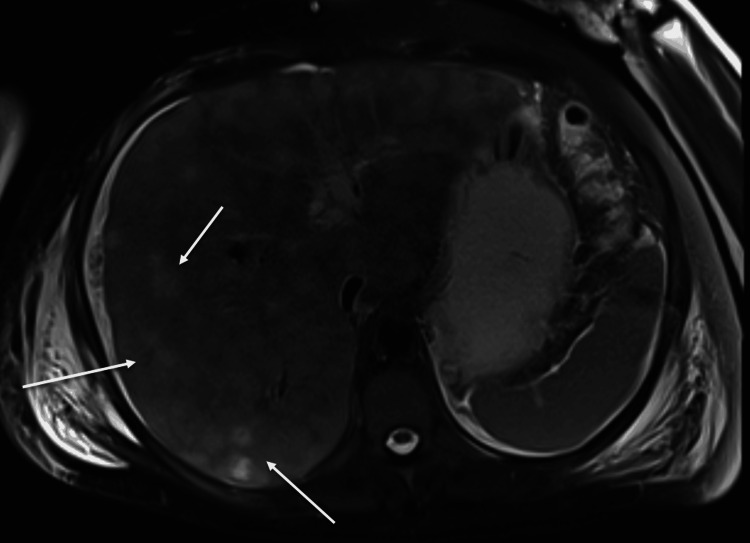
MRI abdomen from case two showing diffuse hepatic metastatic disease and ascites.
